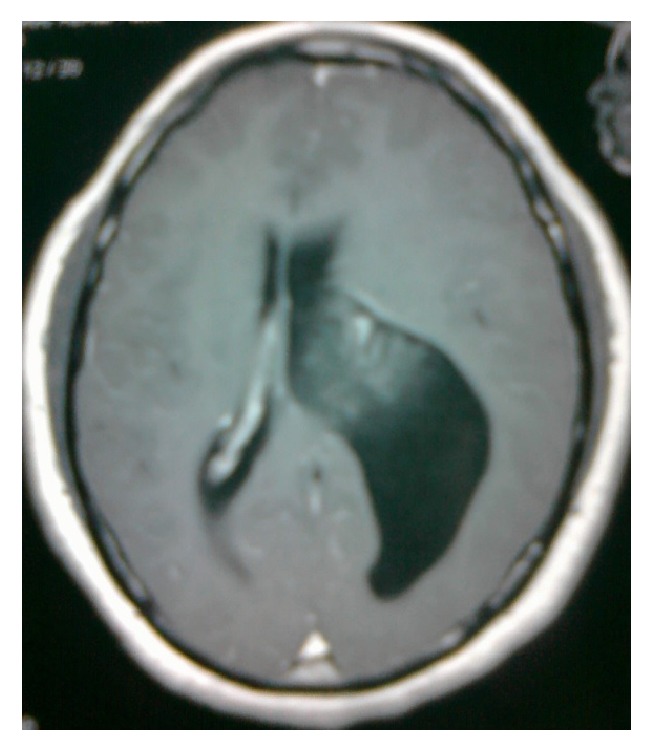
Brilliantly enhancing lesion in left lateral ventricle on MRI.
